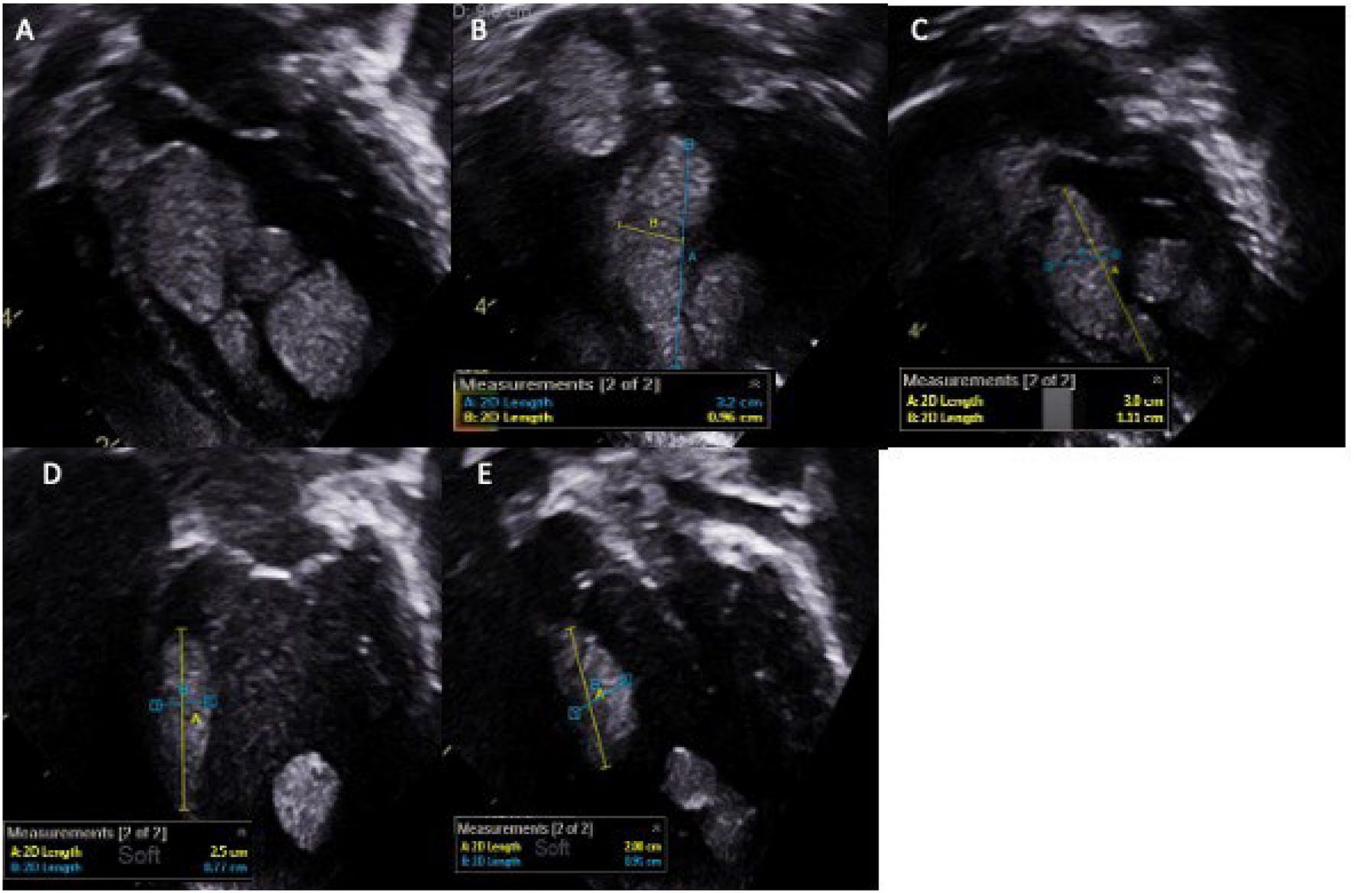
Serial Transthoracic Images in cas 5. A A5C view at 2-weeks, B A4C view at 4-weeks & C A4C view at 12-weeks of Sirolimus therapy showing minimal change in tumour size; D&E A4C & A5C views at 20-months after cessation of mTORi therapy showing 15–30% natural tumour regression
